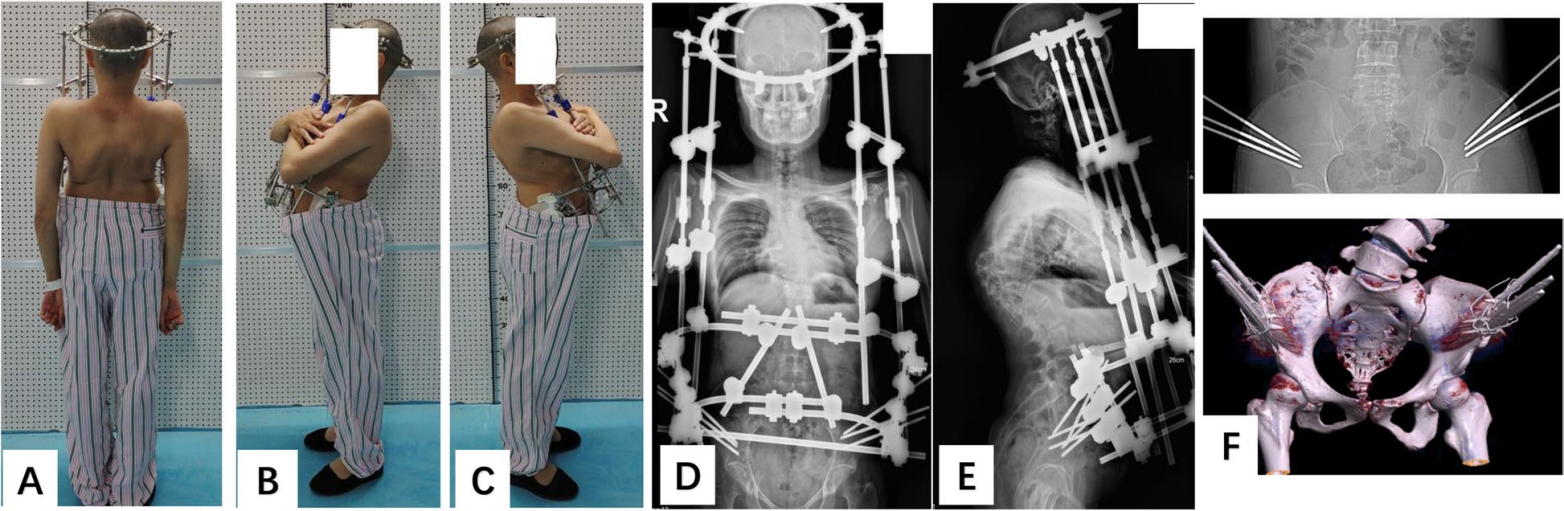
Modified halo-pelvic traction. Posterior (A) and lateral (B, C) view photographs. Standing anteroposterior (D) and lateral radiographs (E). Photographs of the bony pelvis with pelvic pins (F)
